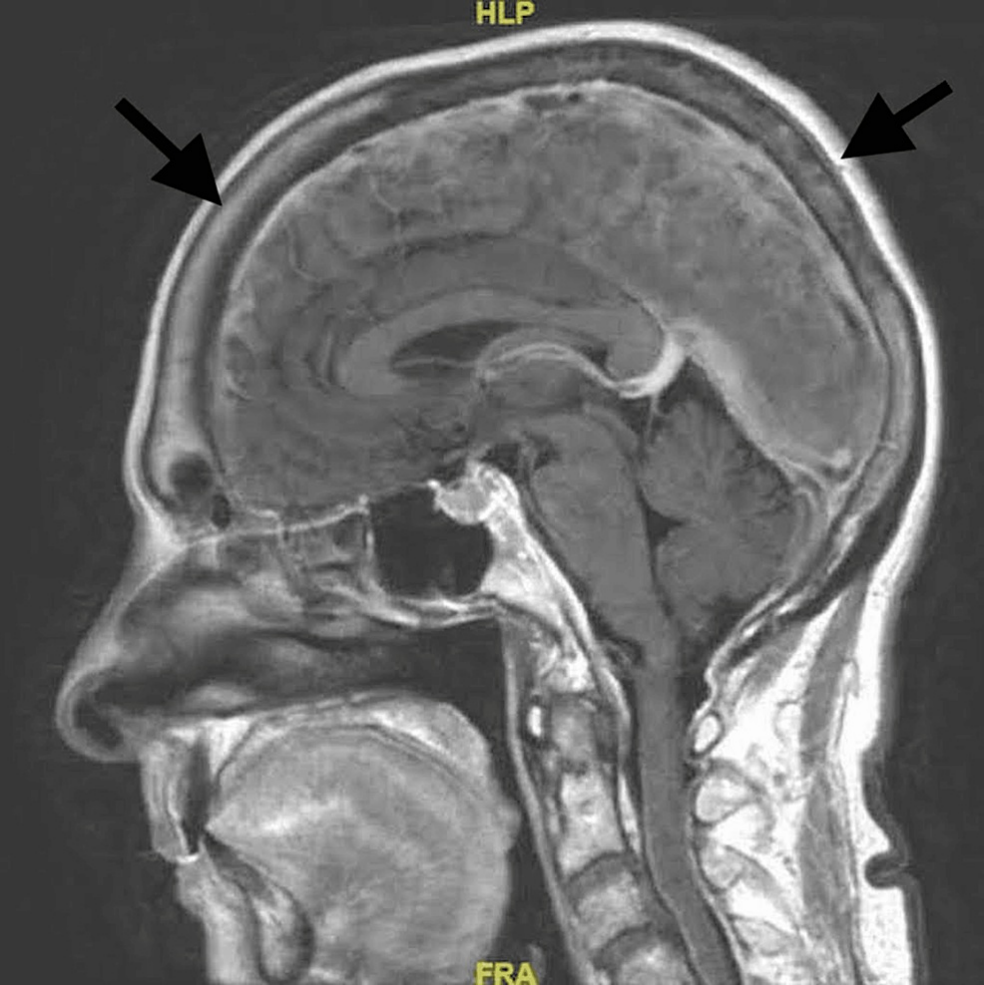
An MRI of the brain (sagittal) T1 FLAIR with contrast Arrows indicate diffuse pachymeningeal thickening that extends into the cervical spine. FLAIR: fluid-attenuated inversion recovery
